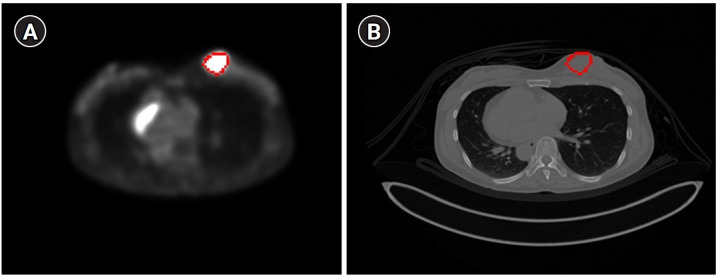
The tumor with a maximum standardized uptake value greater than the 50% threshold displayed on a breast cancer (BC) positron emission tomography image (A) and a BC computed tomography image (B) using the contour method.
